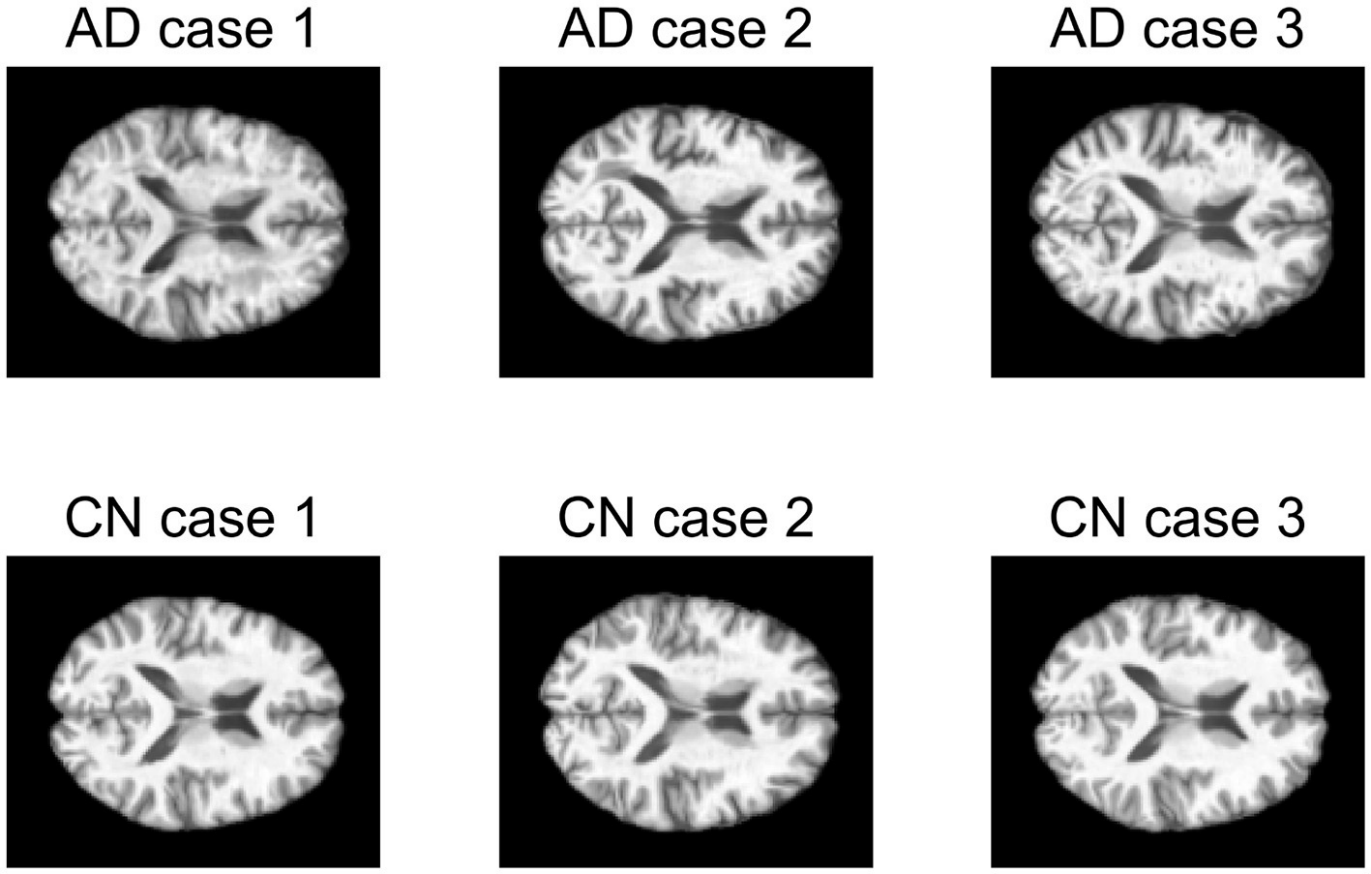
Examples of magnetic resonance imaging (MRI) images of AD patients and CN people.
